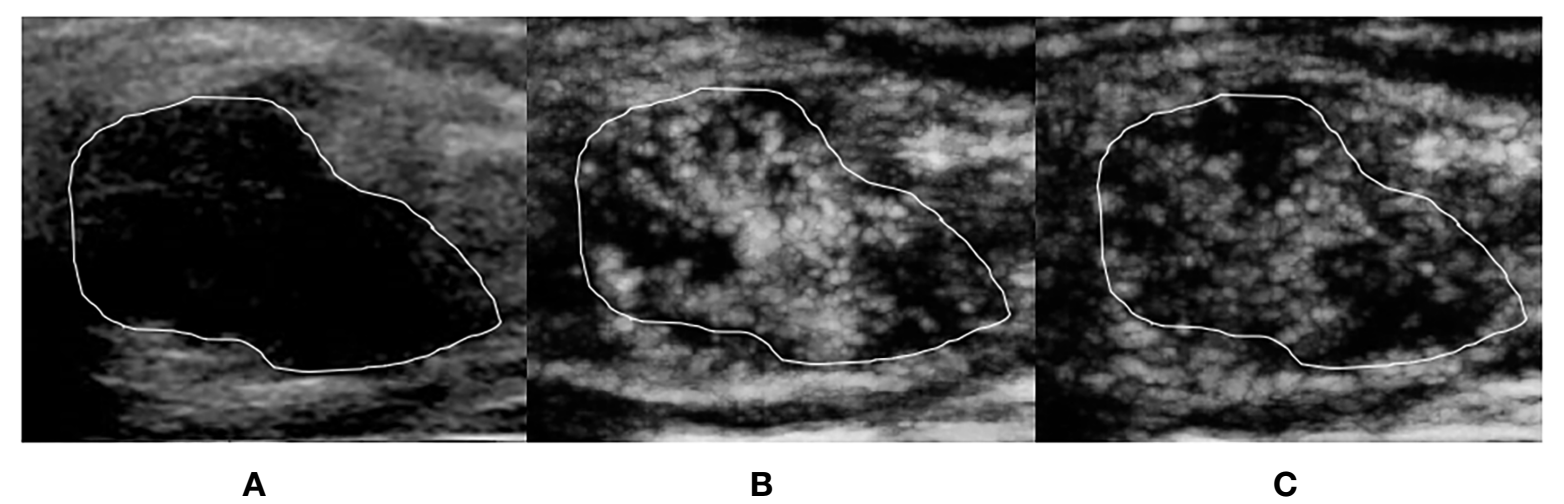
A 39-year-old woman with breast intraductal papilloma proven by pathology. The tumor region is marked in white. (A) Grayscale ultrasound image of breast tumor. (B) The ≤30-s phase: microvasculature morphology shows a tree-like pattern. (C) The >30-s phase: microvasculature morphology shows a tree-like pattern, which is not as clear as the ≤30-s phase.
